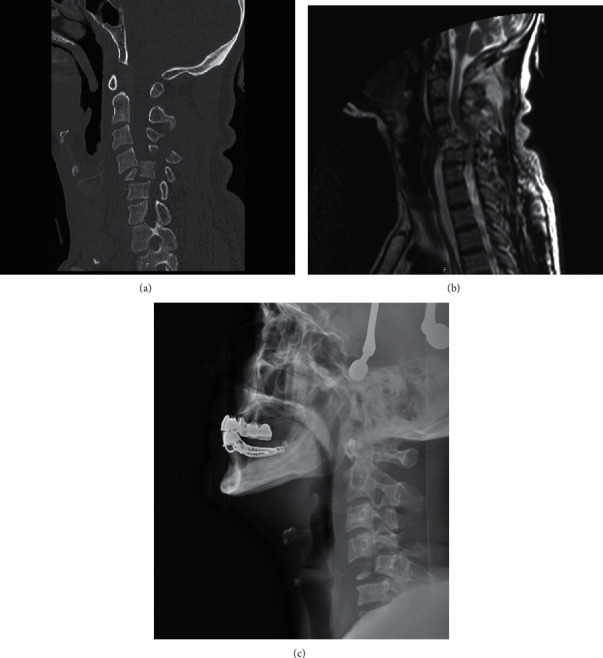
Computerized tomography (a), magnetic resonance imaging (b), and plain radiograph views (c) of cervical spine injury after dive into shallow water.
